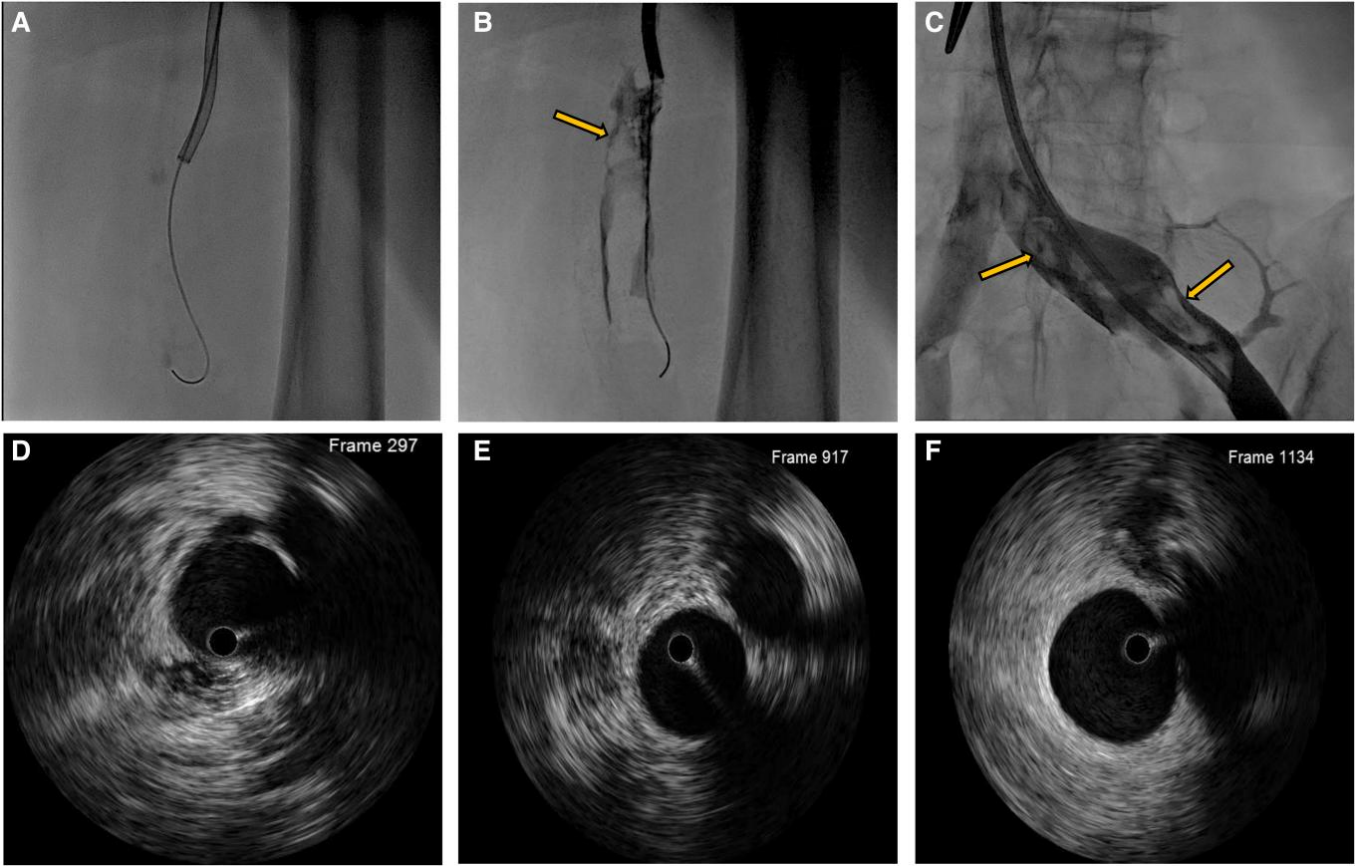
Iliofemoral–popliteal thrombosis and intravascular ultrasound assessment after thromboaspiration. (*A–C*) Contrast venography of the left iliofemoral venous system, highlighting extensive thrombosis. (*D–F*) Intravascular ultrasound images of the popliteal, femoral, and iliac veins following mechanical thromboaspiration, confirming complete resolution of the thrombotic burden and restored vessel patency.
